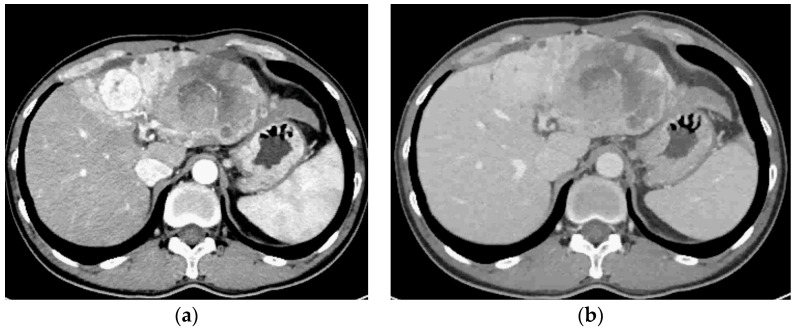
Pre-treatment liver dynamic CT with enhancement at diagnosis of HCC: (a) arterial phase; (b) portal phase.
